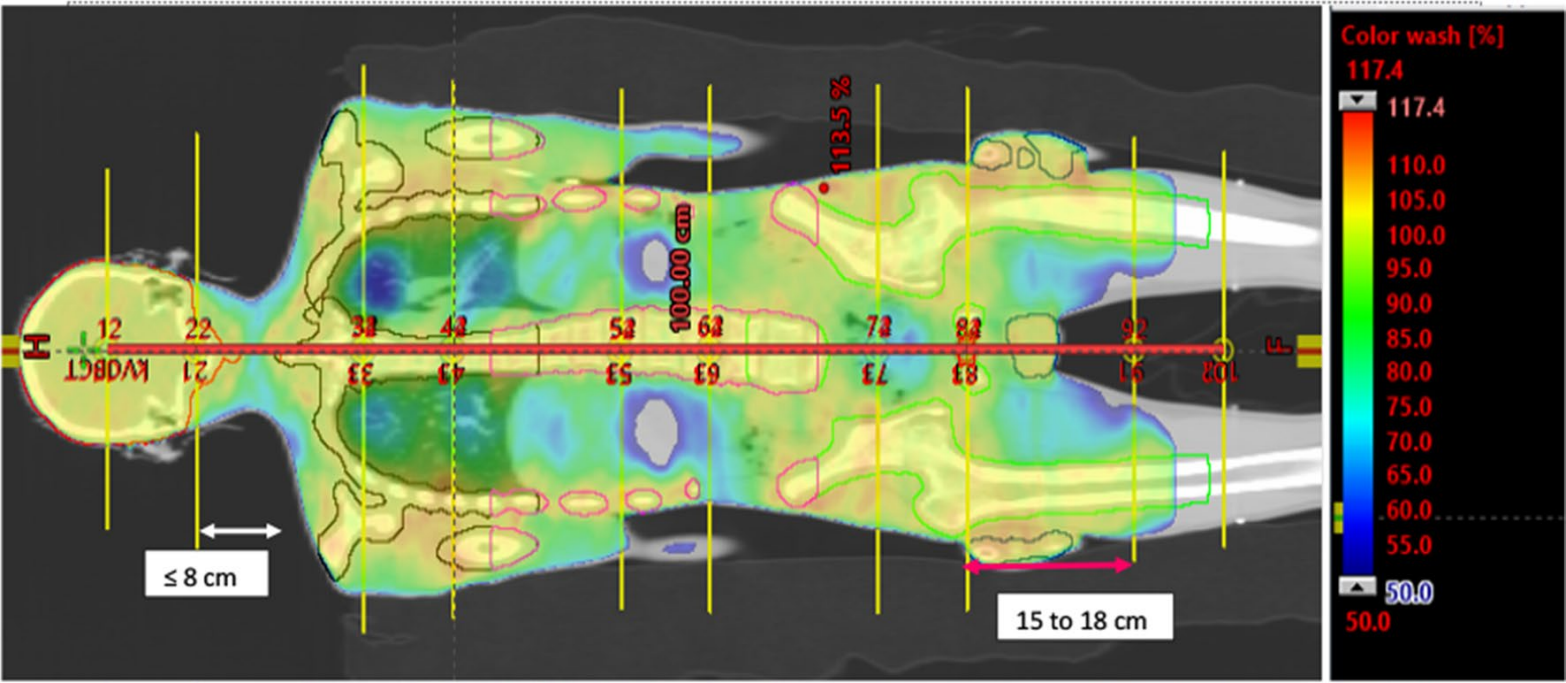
Icocenter and arc placement and dose distribution until 50% of the prescription dose for HFS CT set. Further detail can be found in Additional file 1
